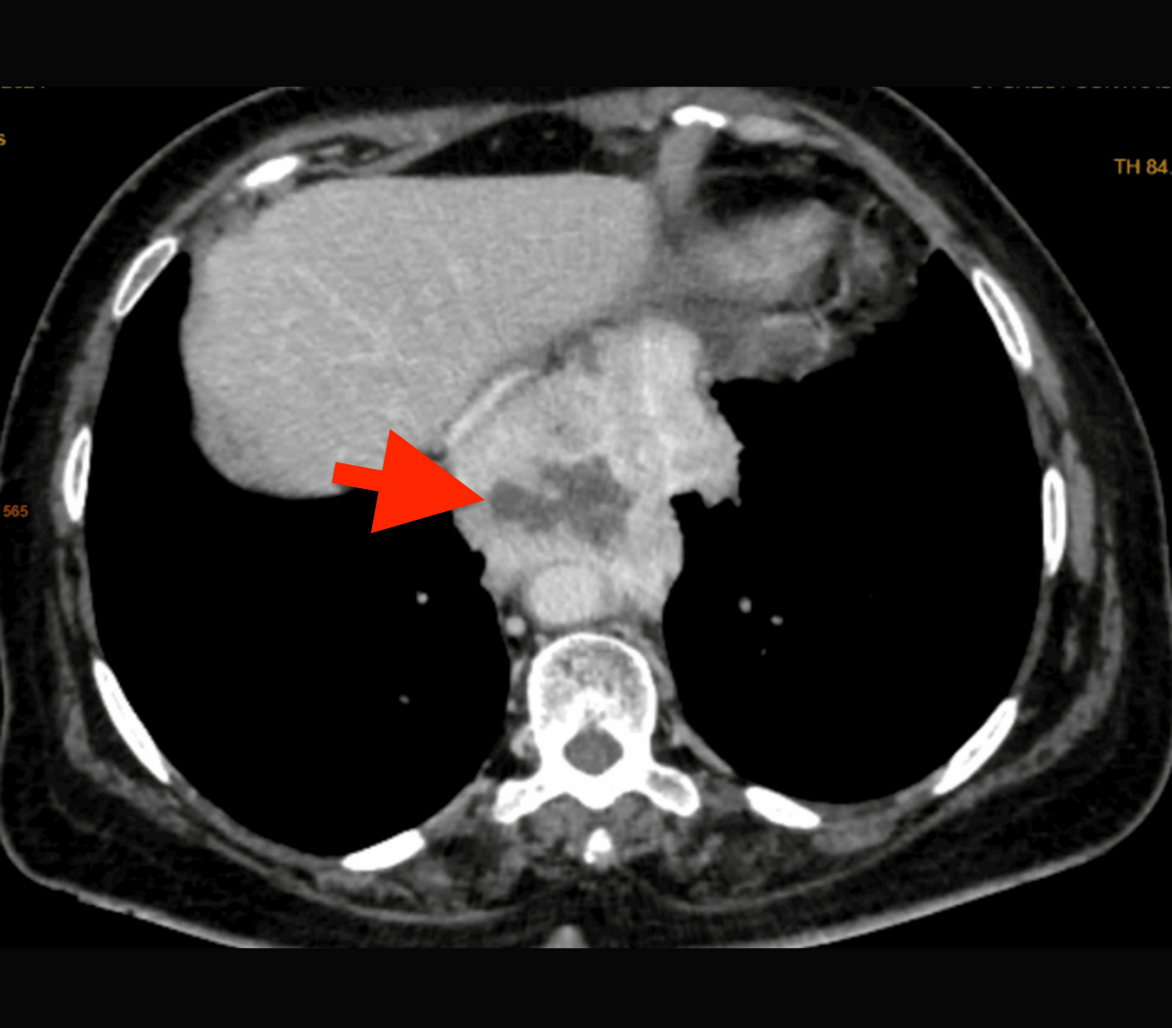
Contrast-enhanced computed tomography; mixed density lesion size 64x84 mm noted in posterior mediastinum showing patchy peripheral post-contrast enhancement with central non-enhancing areas with multiple hyperdense foci with epicenter esophagus. Red arrow: Esophagus.
